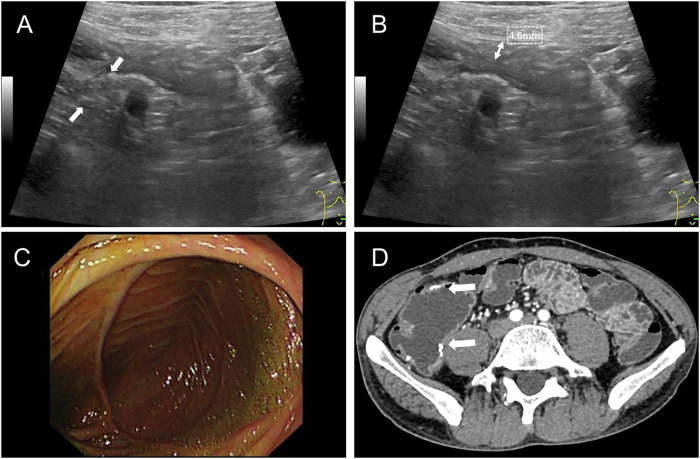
A 39-year-old male patient with CD presented with abnormal thickening of the intestinal wall without HMF. The mesenteric fat was normal, displaying striated, intermixed echoes adjacent to the bowel wall (A, solid arrow), and the thickness of the ileum measured 4.6 mm (B). One year after a partial enterectomy, no ulcers were found during endoscopy (C), and a CT scan showed that the bowel wall at the anastomosis (hollow arrow) was normal (D). CD, Crohn’s disease; HMF, hyperechoic mesenteric fat
